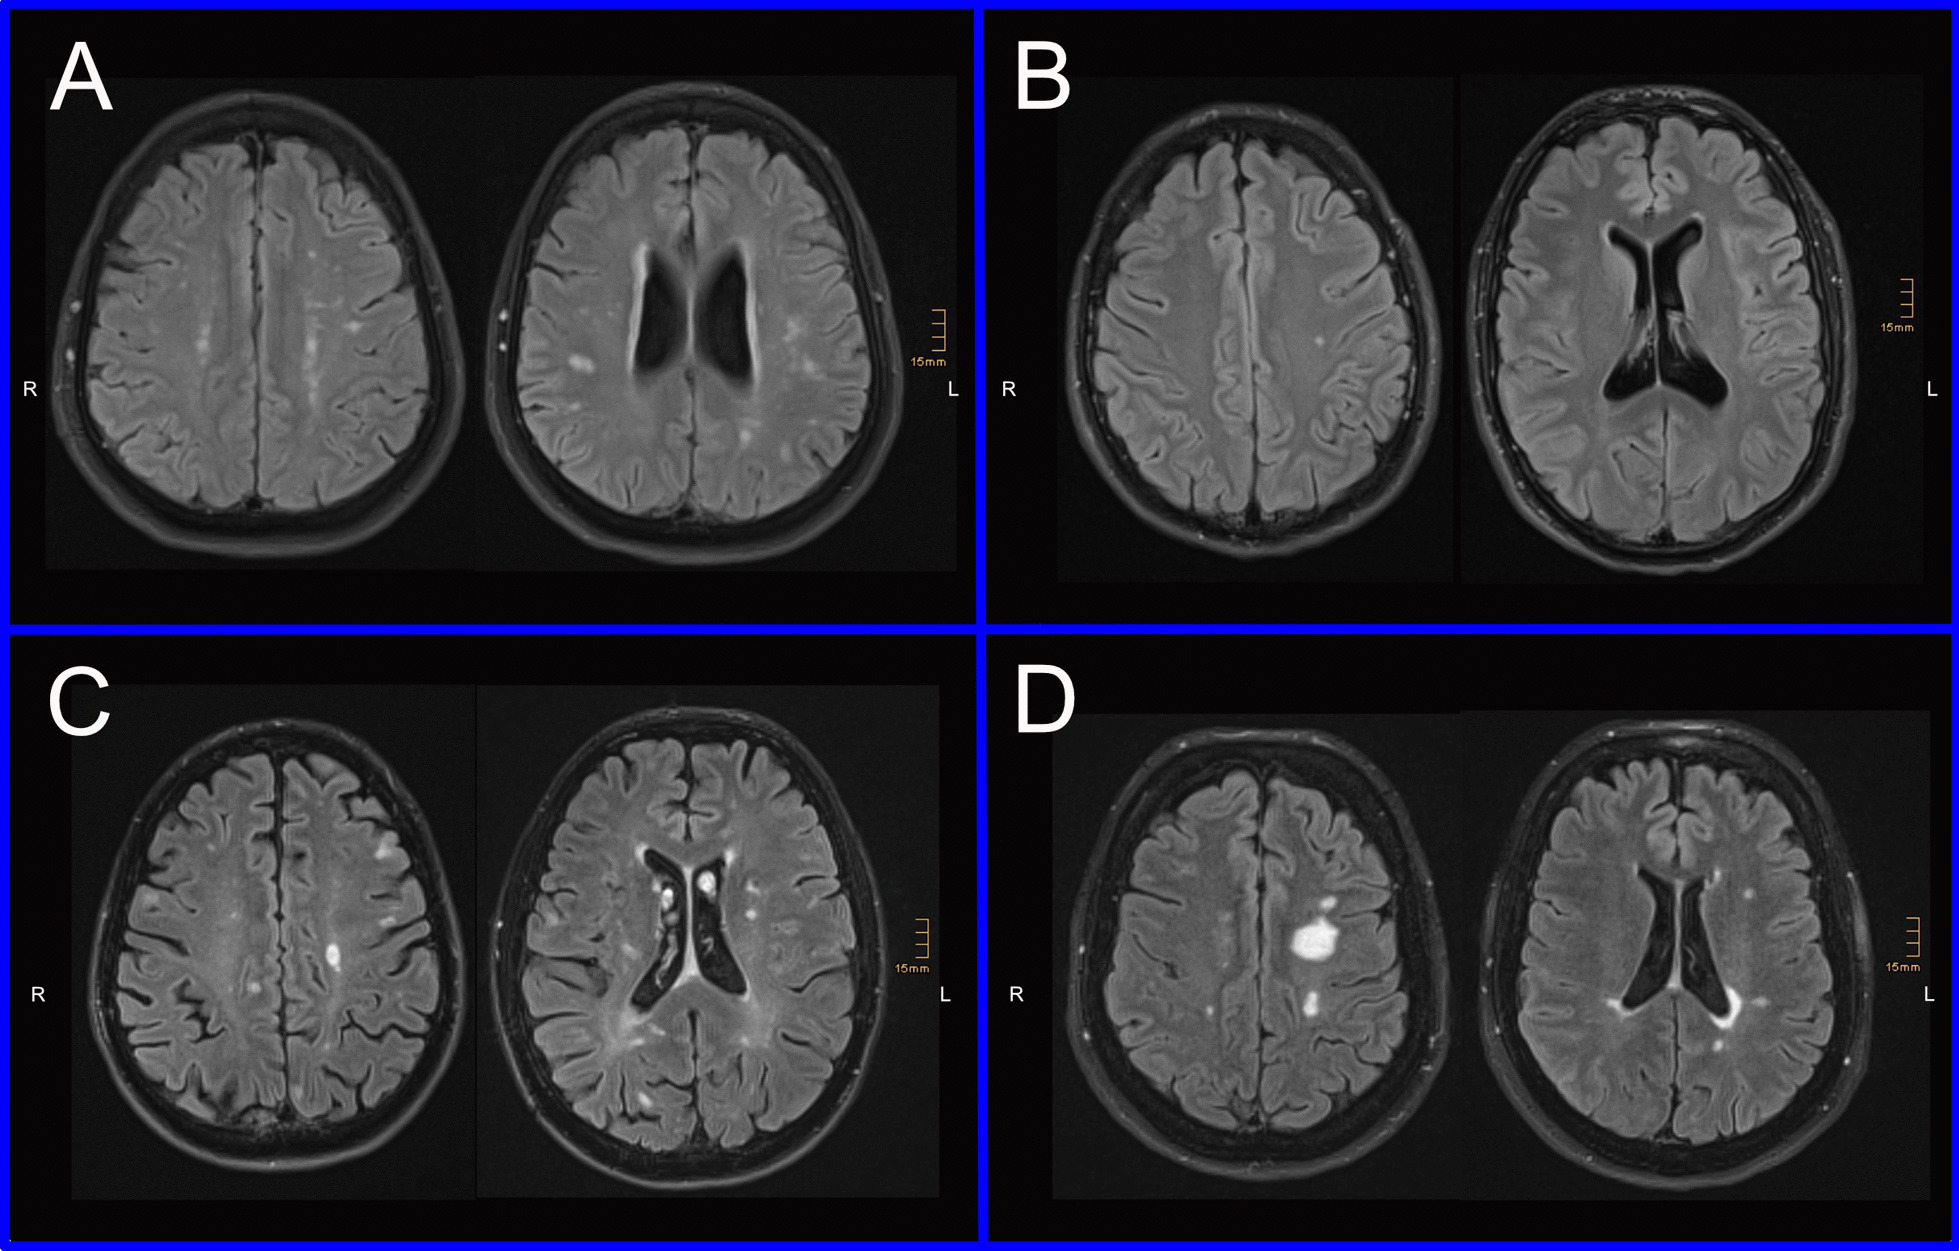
Examples of white matter lesions on FLAIR images. a 65-year-old woman with Fabry disease receiving enzyme replacement therapy; all raters incorrectly classified images as MS. b 31-year-old male patient with Fabry disease receiving enzyme replacement therapy; 5 of 6 raters correctly classified the images as FD. c 36-year-old male patient with relapsing remitting multiple sclerosis (EDSS 3.5, therapy with alemtuzumab at the time of MR scan; 4 of 6 raters correctly classified the images as MS. d 61-year-old female patient with secondary progressive multiple sclerosis (EDSS 6.5, no therapy at the time of MR scan; 5 of 6 raters correctly classified the images as MS
